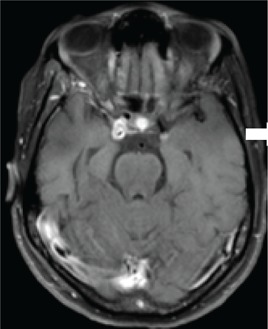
Right internal carotid artery saccular aneurysm with wall enhancement (*arrow*)	Confirmed on CT angiogram
